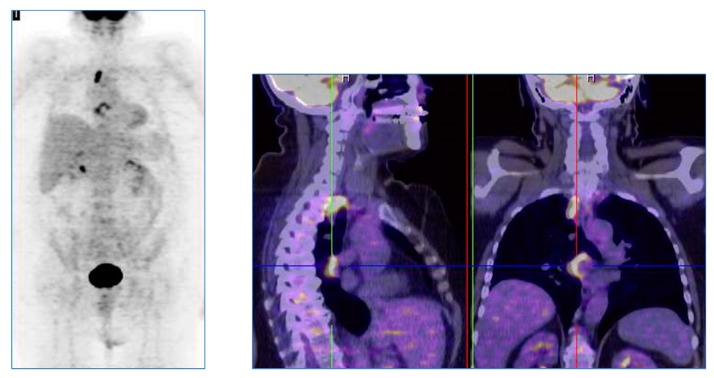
A 59 year-old woman with bladder cancer underwent abdominal contrast-enhanced CT (ceCT) for restaging of disease. CT images were considered completely negative. A positron emission tomography/computed tomography (PET/CT) performed after 3 months, for the persistence of suspicious symptomatology, showed a significant fluoro deoxyglucose uptake in the abdominal lymph nodes and in the liver, compatible with the presence of disease recurrence. The patient was later treated with systemic chemotherapy.
